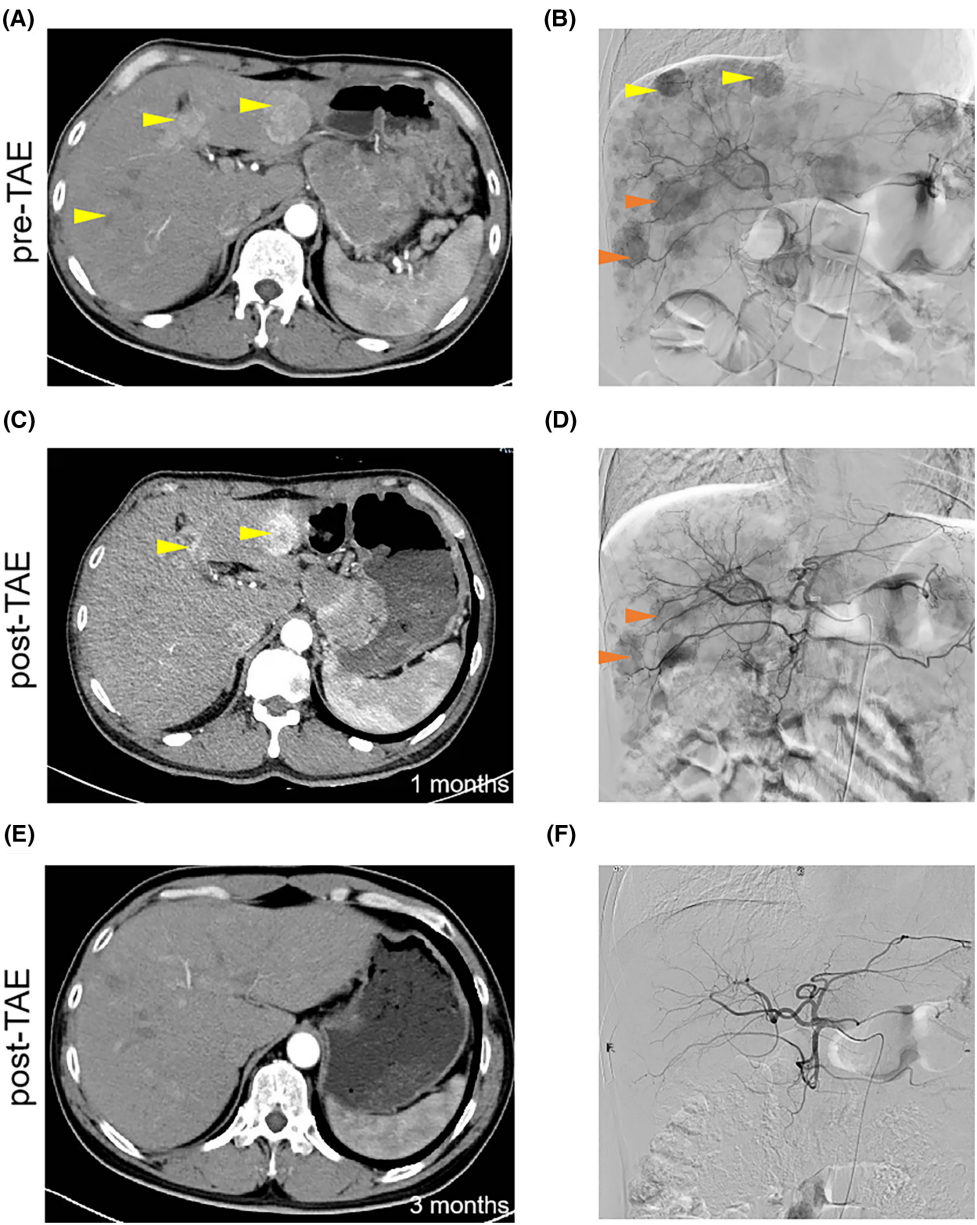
Effect of TAE therapy in a patient with pancreatic NENLM. (A, B) The other case was a patient with type III pancreatic NENLM with hypervascularity evaluated by contrast‐enhanced CT and DSA. (C, D) The main blood vessels of patients with NENLM indicated by the yellow arrows were blocked 1 month later, and the tumour shrank, even including the lesions indicated by the orange arrows. (E, F) All NENLM had disappeared by CT examination 3 months later, and the evaluation of the effect reached CR. The yellow and orange arrows indicated the NENLM lesions. CR, complete response; CT, computer tomography; DSA, digital subtraction angiography; NENLM, neuroendocrine neoplasms with liver metastasis; TAE, transhepatic artery embolization.
